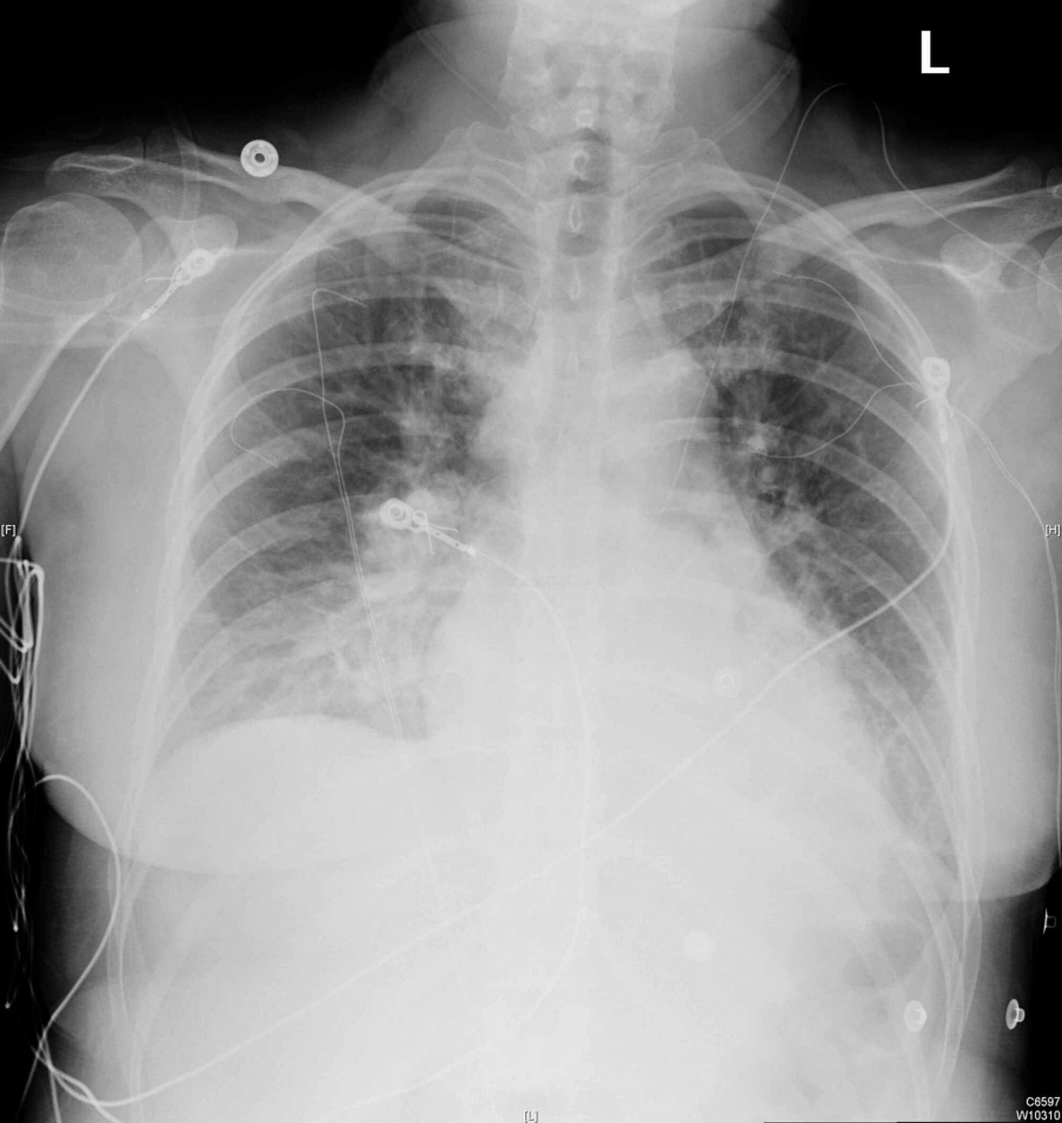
Chest radiograph demonstrating thickened interstitial markings, pulmonary edema, and bilateral pleural effusions
